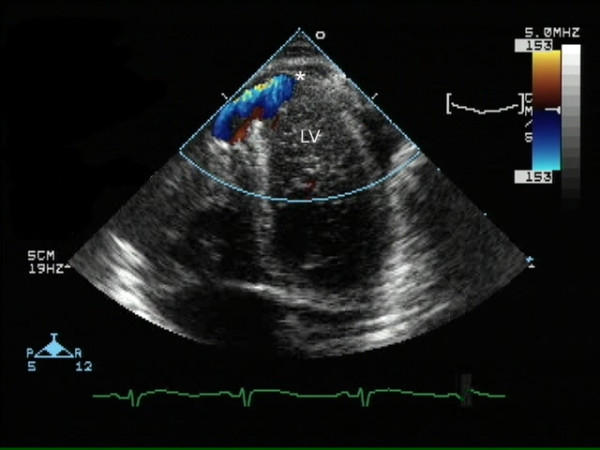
Apical site of the CAF insertion into the LV (*) and the adjacent muscular ventricular septal defect shown by color Doppler.
